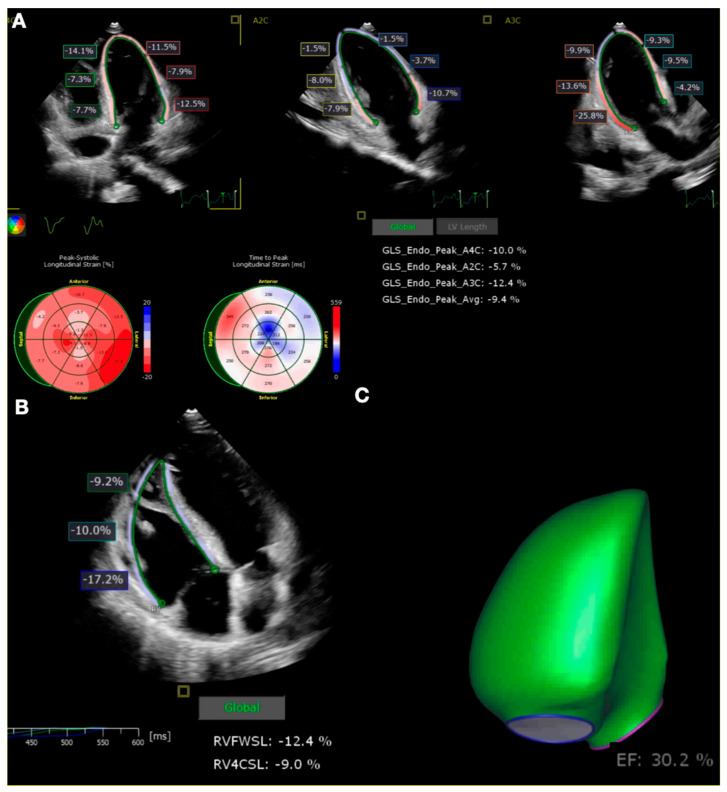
Global longitudinal strain assessment on transthoracic echocardiography. Global longitudinal strain of left ventricle on apical four-chamber, apical two-chamber, and three-chamber views (A), global longitudinal strain of right ventricle (B), and 3D reconstruction of right ventricle ejection fraction (C).
